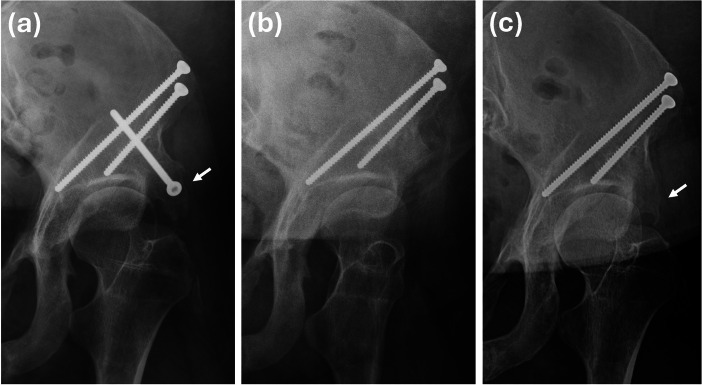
A case example of a 15-year-old female who underwent periacetabular osteotomy with retrograde screw fixation. a) Two years and four months post-surgery, she developed type 4 diffuse heterotopic bone formation. b) The retrograde screw and heterotopic bone were subsequently removed. Unfortunately, she experienced gradual recurrence of heterotopic bone formation after the excision. She underwent conservative treatment, and c) an anteroposterior radiograph taken four years after excision demonstrated a stationary condition of the heterotopic bone.
